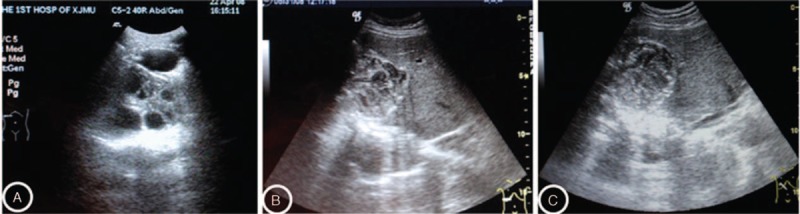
Ultrasound images of the CE lesions obtained from the baseline period (1A), 3 months (1B), and 6 months (1C) after administration of L-ABZ, respectively. The results indicated that complete response was noted at 6 months after administration of L-ABZ. CE = cystic echinococcosis, L-ABZ = liposomal albendazole.
